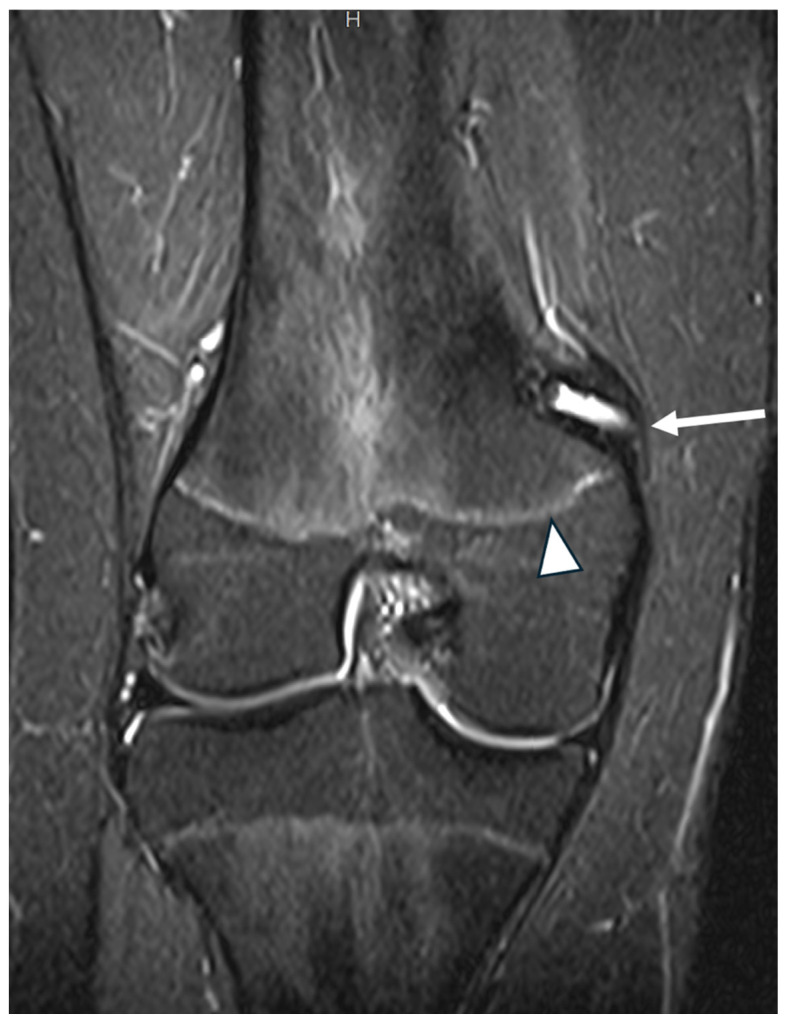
Coronal MRI of the distal femur in an adolescent. The femoral tunnel for the passage of the semitendinosus graft was created superior to the distal femoral physis (arrowhead) in a proximal direction to avoid physis penetration and fixed with an absorbable screw (arrow).
